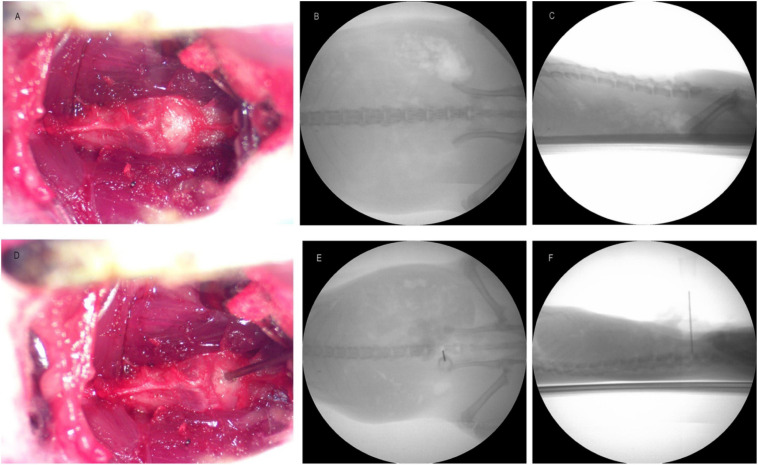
Panel (A) is the image of intervertebral disk before puncture. Panels (B,C) are the X-ray images of anterior and lateral position before puncture. Panel (D) is the picture of intervertebral disk during puncture. Panels (E,F) are the pictures of X-ray at the time of puncture.
